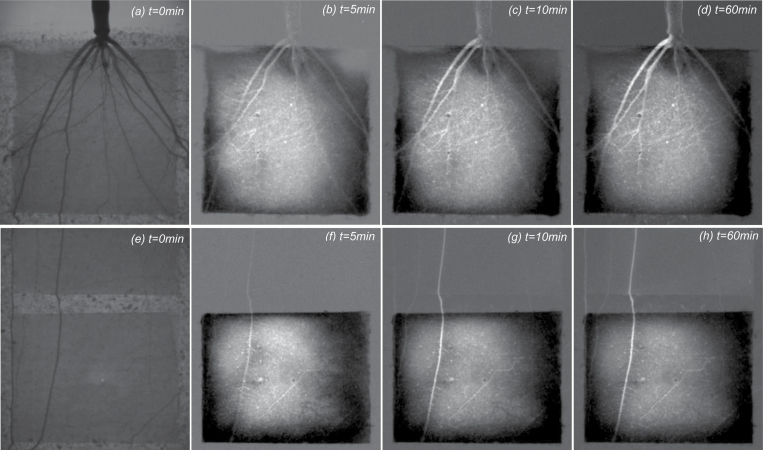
Neutron radiographs of deuterated water (D_2_O) injection in the upper (a–d) and lower (e–h) compartment during the day. (a) The sample before injection (*t*=0). (b–d) The difference between the actual radiograph at time *t* and that before injection (*t*=0). Brighter colors in (b–d) indicate a lower neutron attenuation and higher D_2_O:H_2_O ratio. Conversely, the dark areas show accumulation of H_2_O after D_2_O injection. After injection, the crown roots turned bright immediately, which indicated that D_2_O had entered them faster than the seminal roots and their laterals. (e) The sample before injection. (f–h)The difference between the actual radiograph at time *t* and that before injection (*t*=0). The compartment included the distal parts of a crown root where the laterals had not emerged yet. In contrast to the seminal roots, the crown roots were able to take up water from their most distal part.
